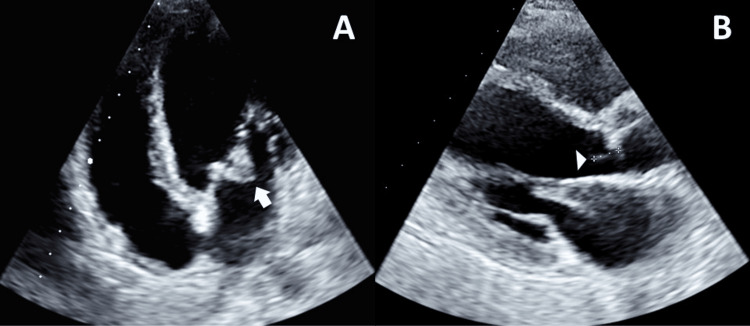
Transthoracic echocardiogram: (A) apical view showing a large vegetation on the mitral valve (arrow) and (B) parasternal long axis view showing a linear vegetation on the aortic valve (arrowhead)
